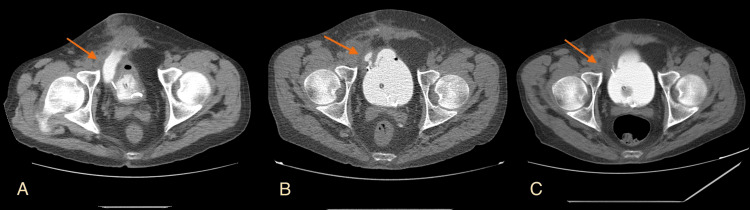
Urine leak after Politano-Leadbetter ureteric reimplantation Axial multidetector computed tomography (MDCT) urography images of the first (A), second (B), and third (C) postoperative months of the ureteric reimplantation show the residual leakage and gradual improvement along with conservative management (red arrows). Image source: Department of Radiology, Ippokrateio General Hospital of Thessaloniki. Permission obtained for reproduction.
